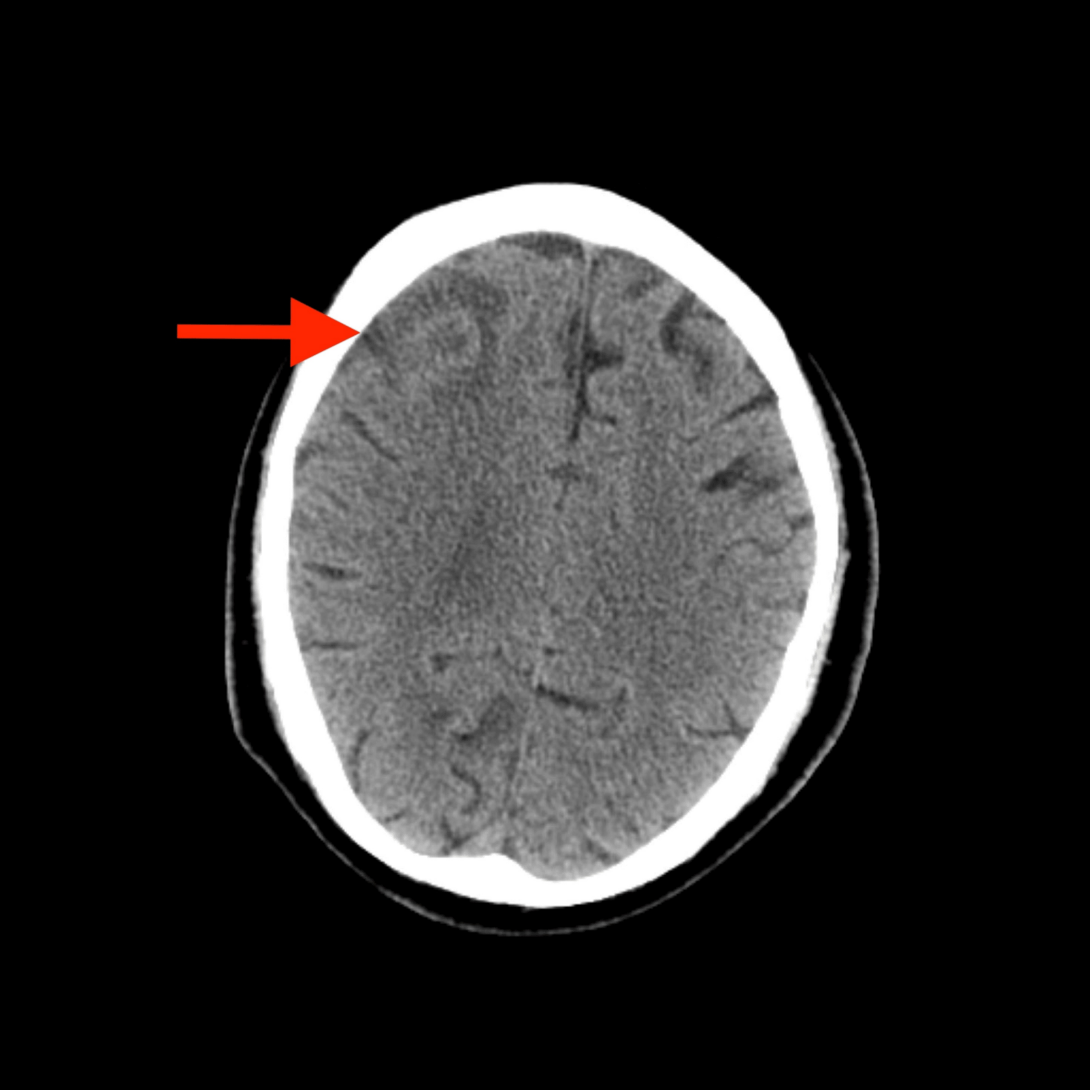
Non-contrast CT of the brain showing a right frontal mass with perilesional edema Axial view demonstrating a mass (red arrow) in the right frontal lobe, exhibiting surrounding vasogenic edema.
